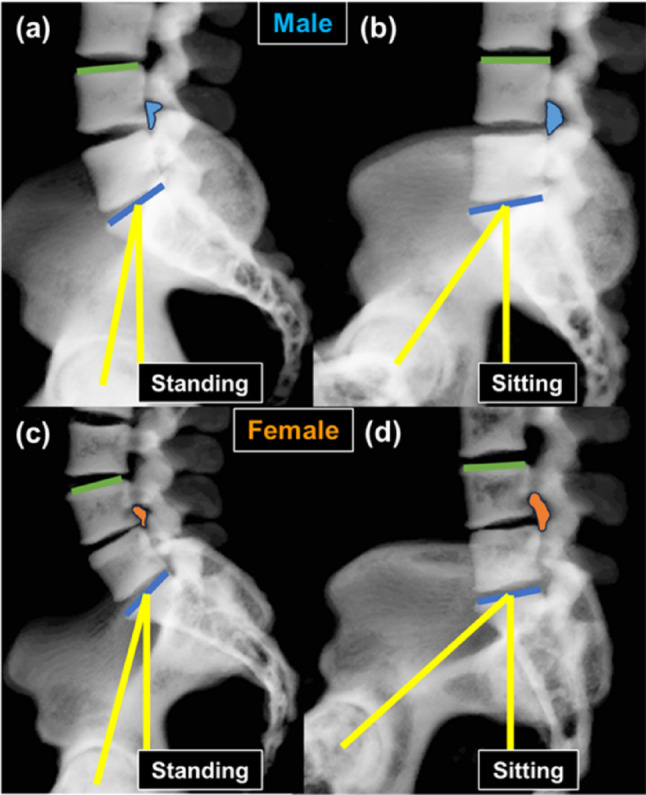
Representative cases demonstrating posture-related changes in spinal alignment and intervertebral foramen area in young adults. a Standing whole-spine CT of a 32-year-old male. b Sitting whole-spine CT of the same 32-year-old male. c Standing whole-spine CT of a 38-year-old female. d Sitting whole-spine CT of the same 38-year-old female. Solid yellow lines indicate pelvic tilt (PT), defined as the angle between the vertical axis and the line connecting the midpoint of the S1 endplate to the center of the femoral heads. The solid blue line on S1 indicates sacral slope (SS), and the angle between the green line on L4 and the blue line on S1 represents L4–S lumbar lordosis. The filled shape represents the bony boundary area (BBA) of the intervertebral foramen (blue in the male case; orange in the female case)
